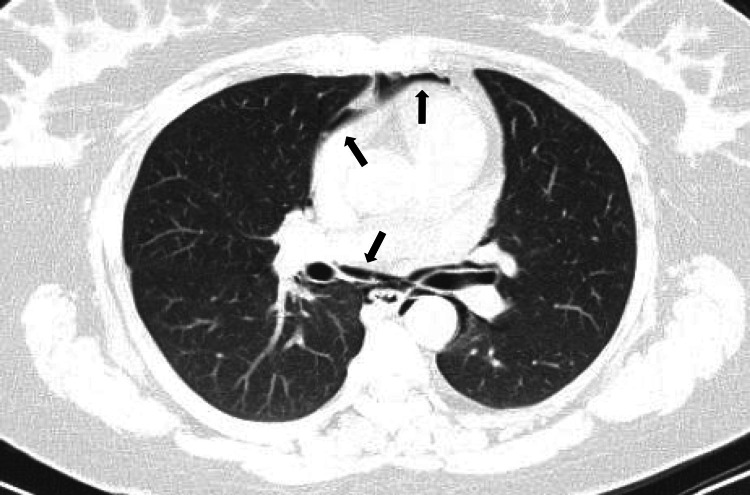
Transverse chest CT scan showing moderate pneumomediastinum (arrows)
